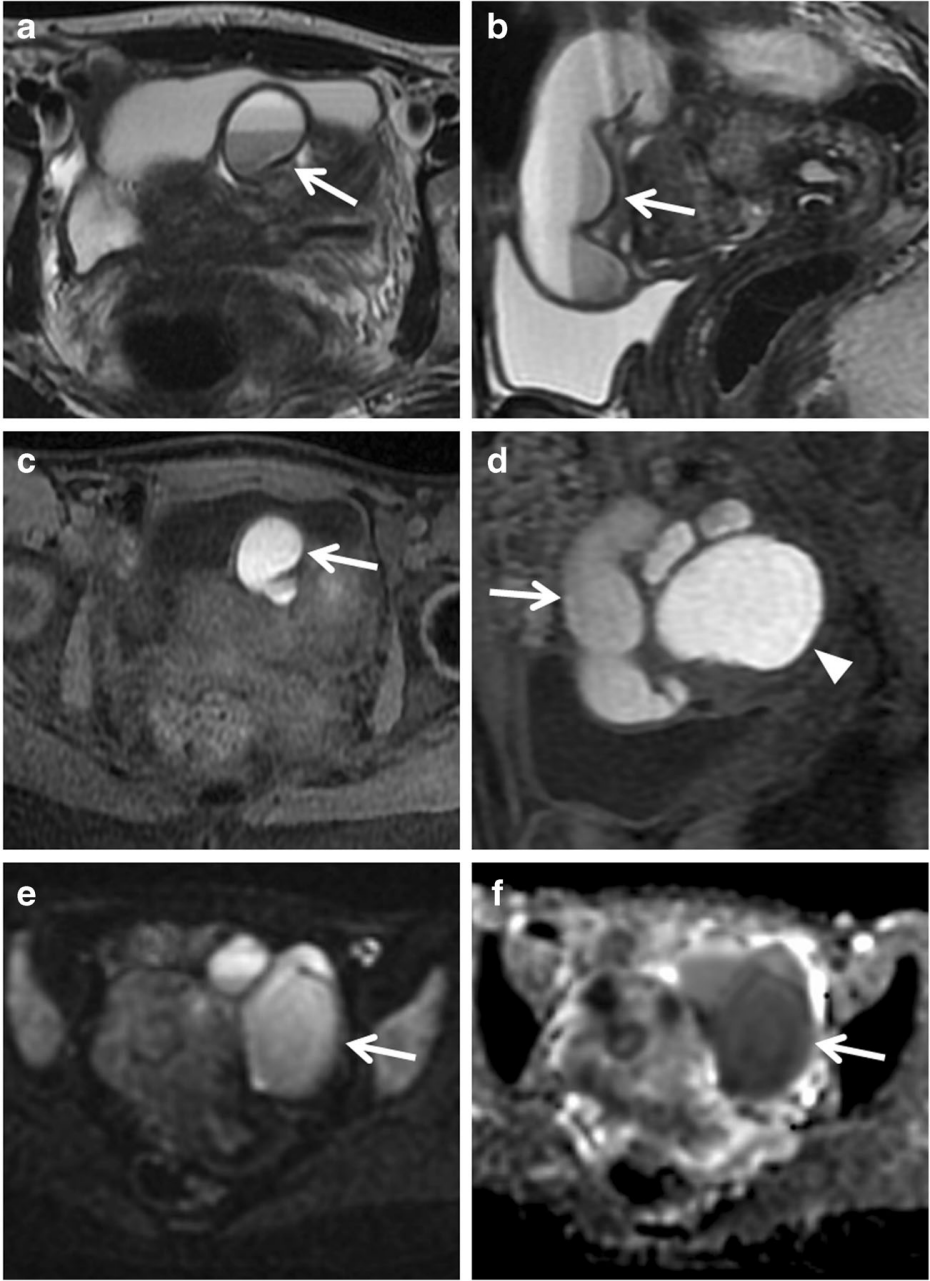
Hematosalpinx in a 35-year-old woman with endometriosis. (a) Axial and (b) sagittal T2-weighted images show a tortuous, tubular structure with internal fluid-fluid level (white arrows) in the left adnexa. There are incomplete effaced mucosal and submucosal plicae along the tubal wall. (c) Axial and (d) sagittal fat-suppressed T1-weighted images show a distended fallopian tube with high signal intensity content (*white arrows*), a finding consistent with hematosalpinx. Note an endometriosic cyst in the omolateral ovary (*arrowhead*). (e) Axial DW image (b = 800 s/mm^2^) and (f) corresponding ADC map show that the lesion’s content demonstrates restricted diffusion with high signal intensity (*white arrow*) on DWI image and low signal intensity (*white arrow*) on ADC map
